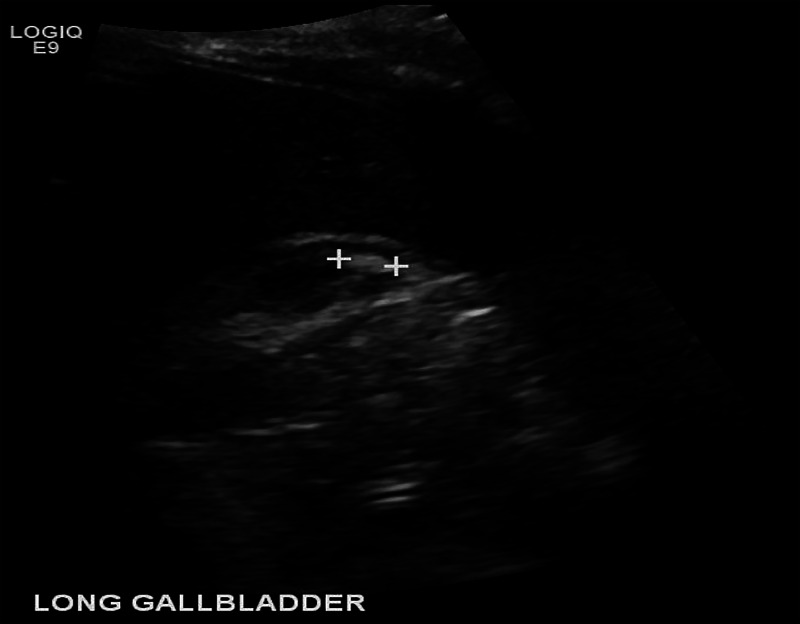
Abdominal ultrasound Preoperative ultrasonography highlighting the assumed non-mobile gallstone (between the plus signs) within the gallbladder fundus.
